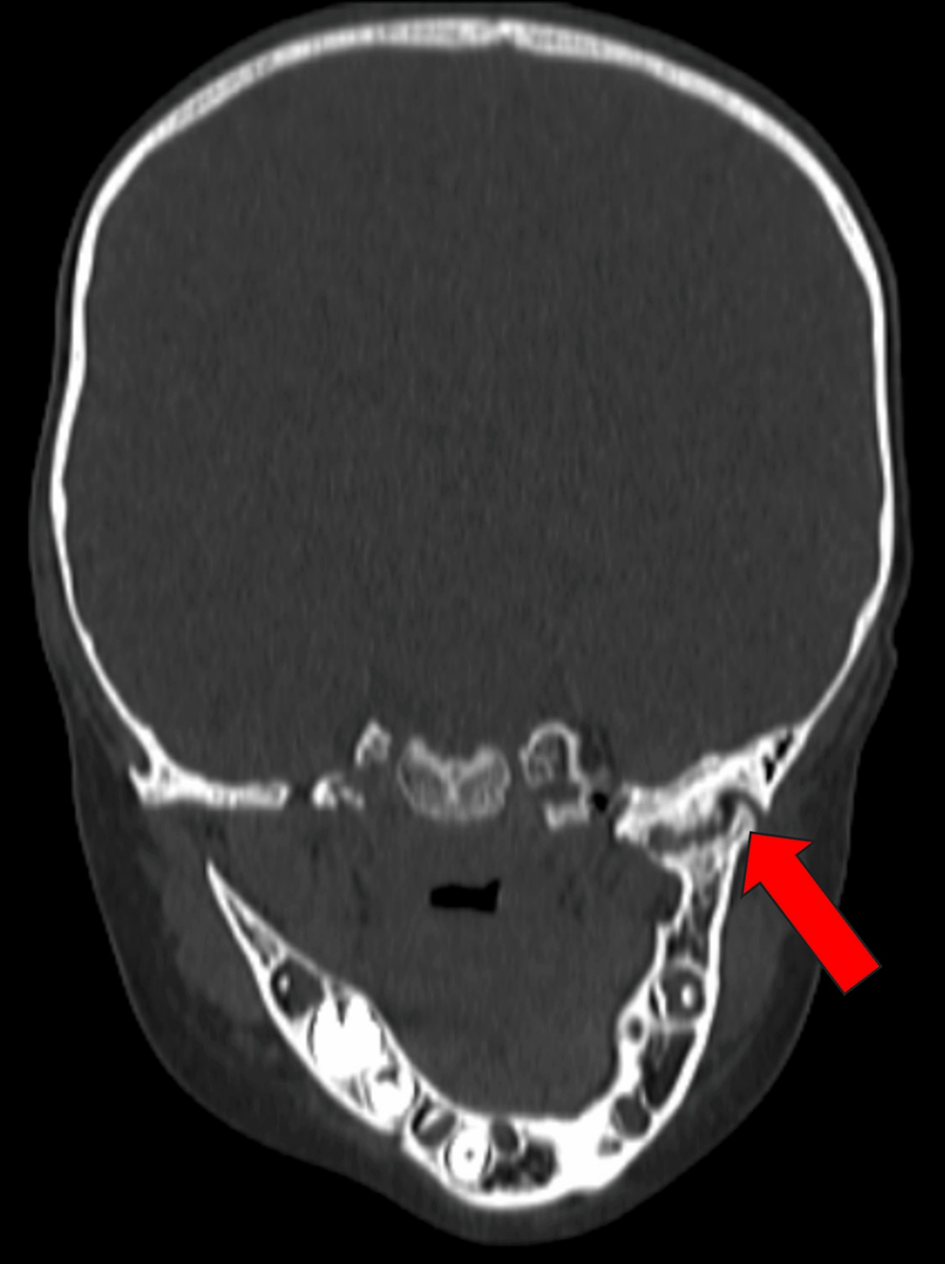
Coronal CT bone window of TMJ showing TMJ ankylosis (red arrow). CT: computed tomography; TMJ: temporomandibular joint
